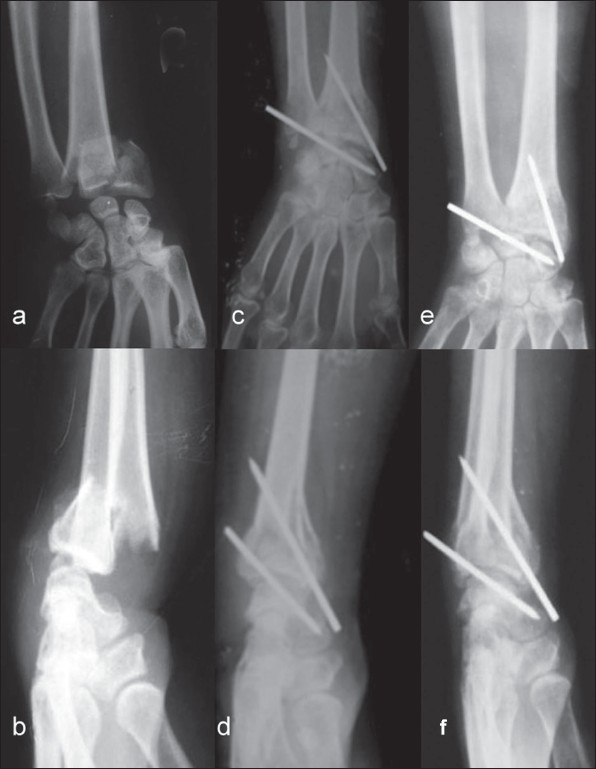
Pre operative anteroposterior and lateral (a, b) xray of the left wrist shows comminuted intraarticular fracture distal end radius (Melon's type-II) with fracture ulnar styloid. Immediate post operative and anterioposterior and lateral X-rays (c, d) and 6 weeks follow-up anteroposterior and lateral X-rays (e, f) shows evidence of union, maintenance of alignment of DRUJ, radial length, normal radial tilt.
